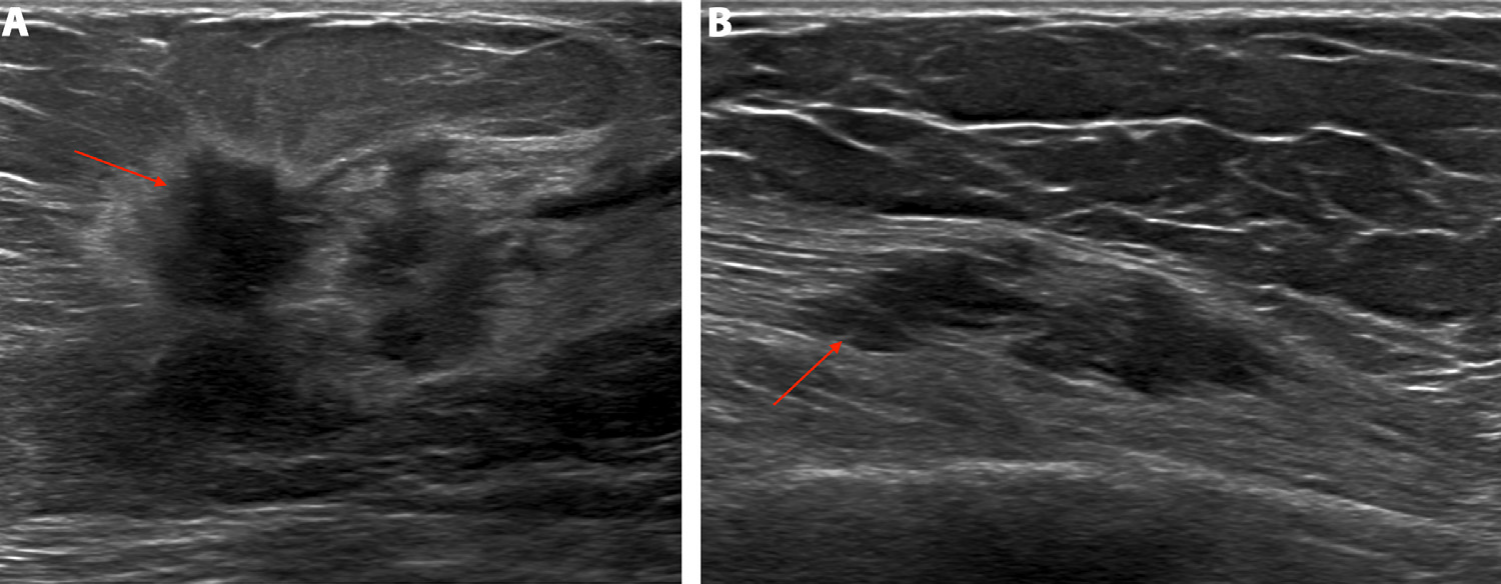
(A) Diagnostic ultrasound of the right breast. At the 10:00 position, with the probe 1 cm from the nipple, an irregularly-shaped hypoechoic mass with spiculated margins is visualized measuring 2.4 × 2.6 × 1.6 cm (arrow). (B) Diagnostic ultrasound of the right breast. At the 11:00 position, with the probe 9 cm from the nipple, a heterogeneously hypoechoic mass within the right pectoralis muscle is visualized measuring 2.9 × 2.6 × 0.8 cm (arrow).
